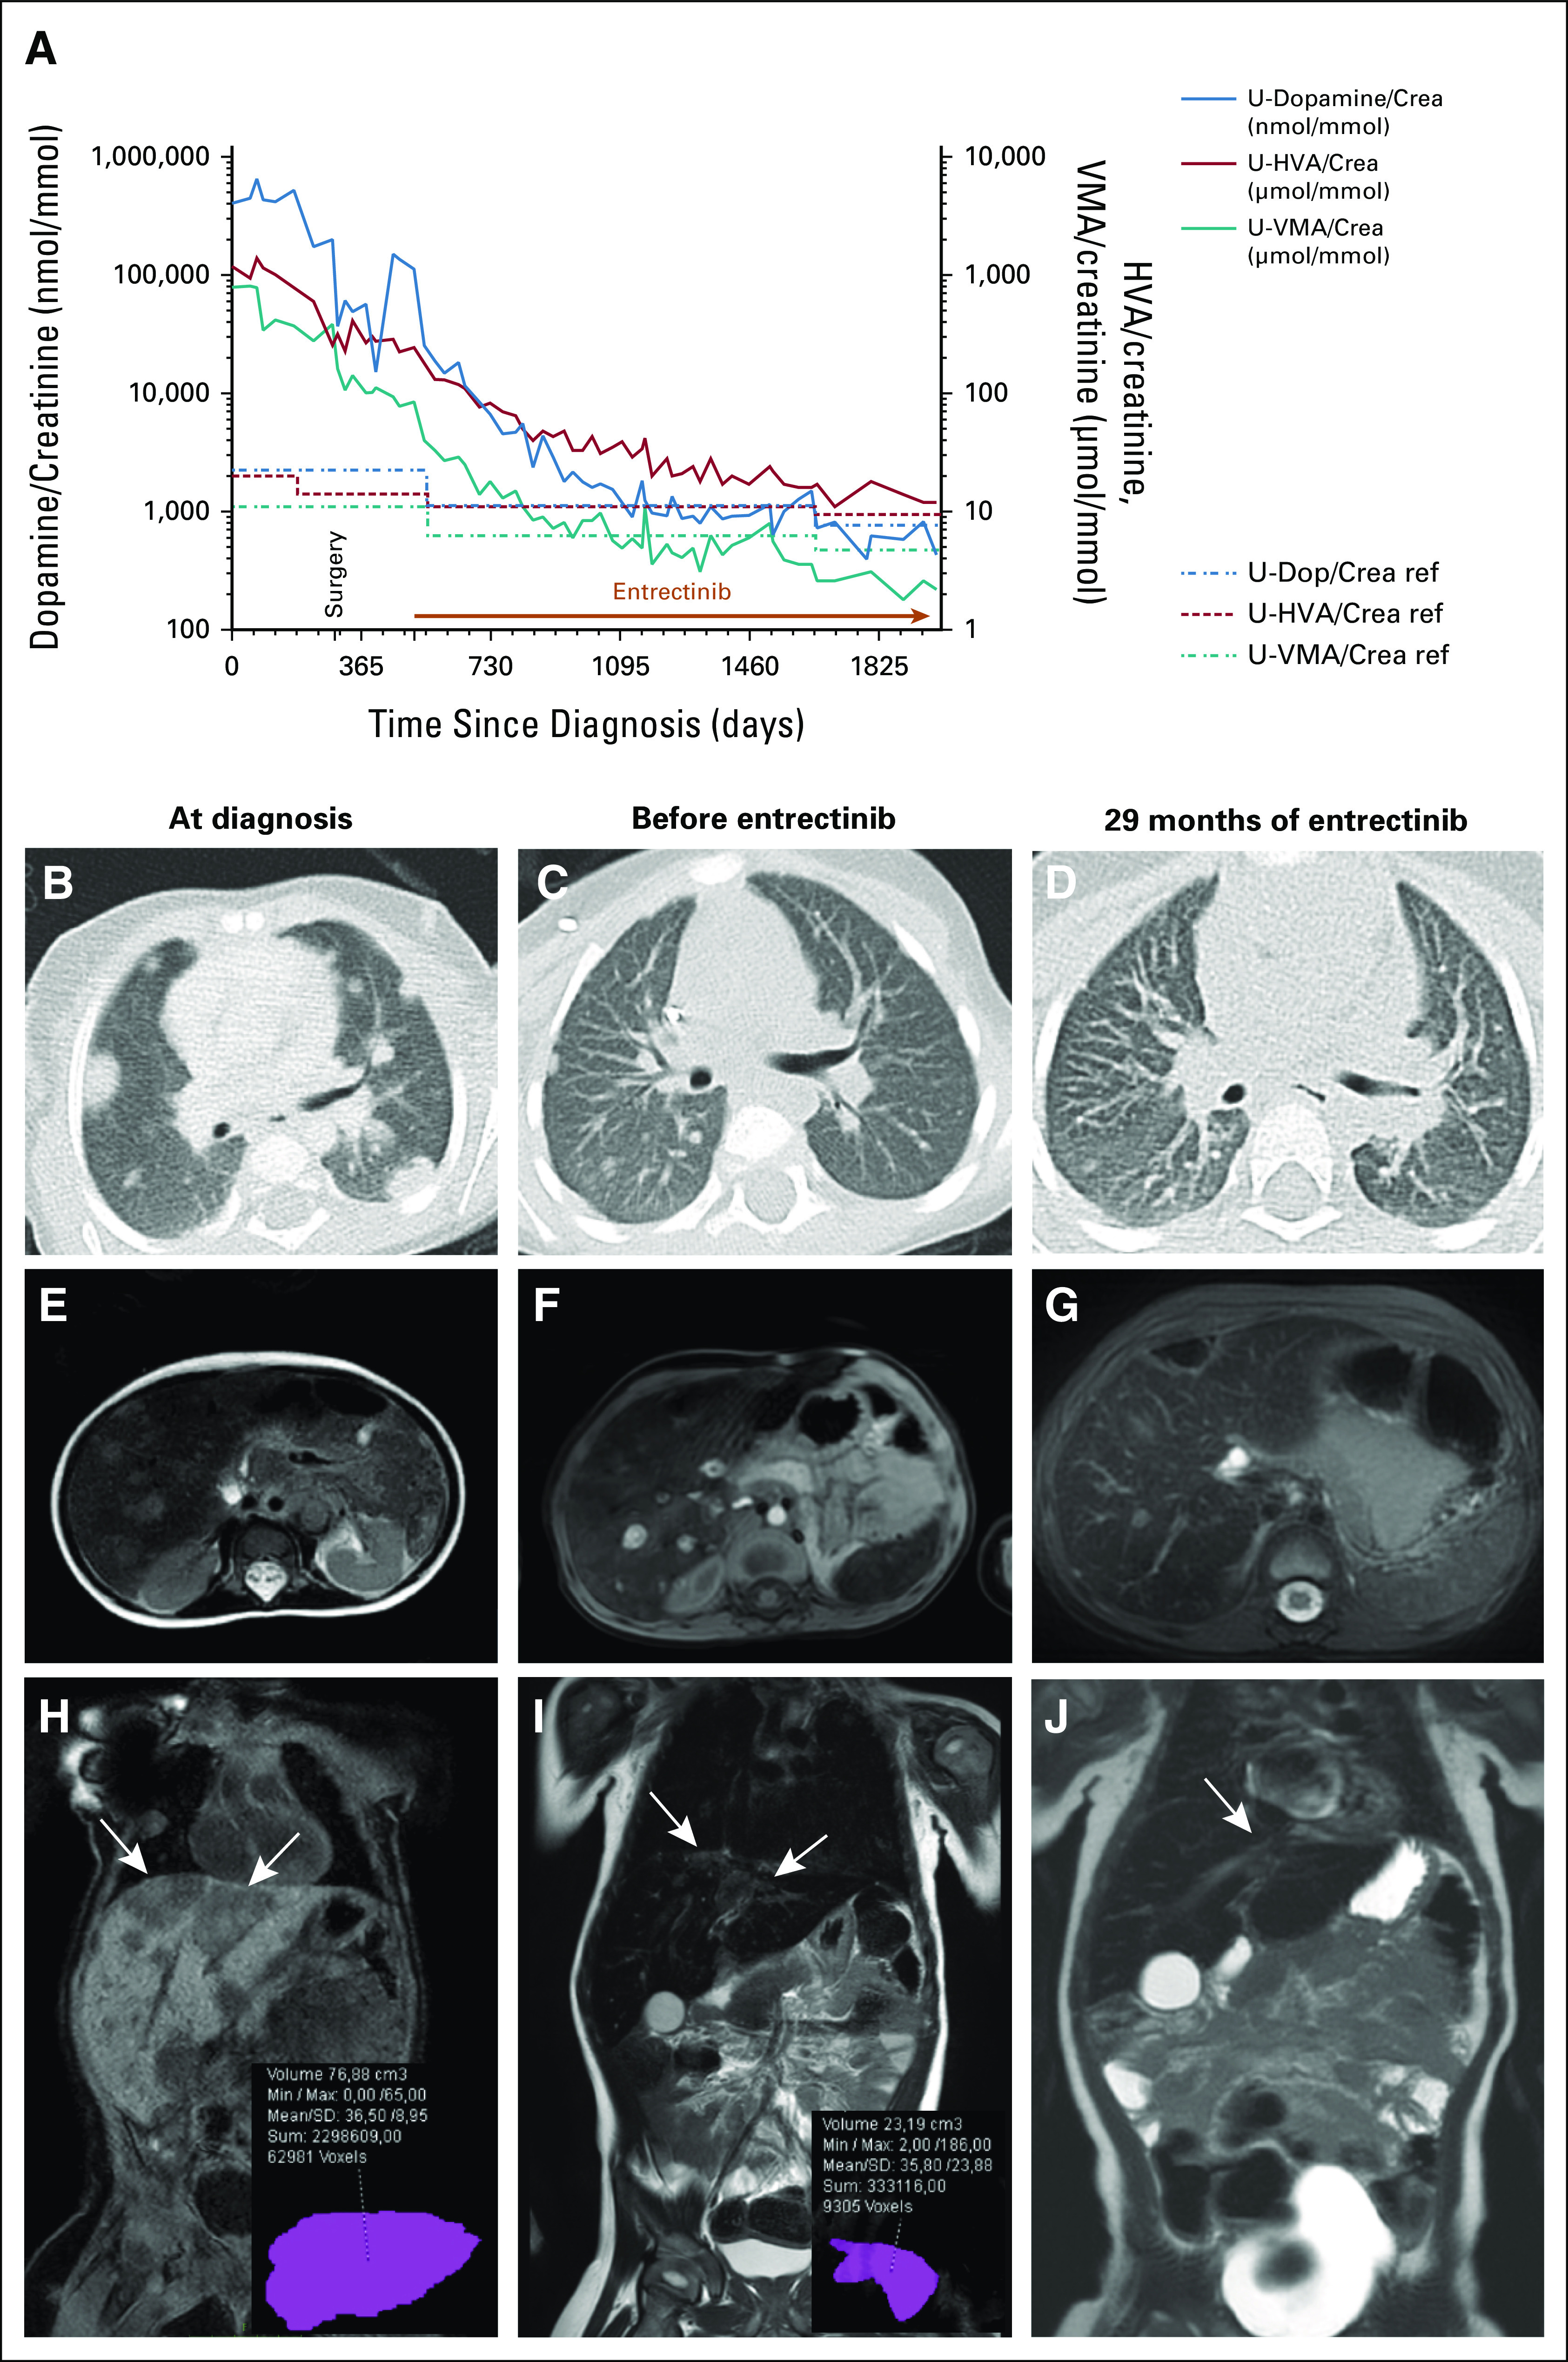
Response to treatment. (A) Urine catecholamine metabolites, logarithmic scale. Catecholamine metabolites (dopamine in blue, HVA in red, and VMA in teal) as NB markers in the patient's urine (molar concentrations/creatinine concentration). Dashed lines represent age-specific reference values. At start of entrectinib treatment, the urine dopamine level was still elevated 50-fold beyond the reference level. CT scans of the lungs showing (B) extensive metastasis at diagnosis, (C) decreasing size and number of metastases after multiple courses of chemotherapy, and (D) further involution after 29 months of entrectinib therapy. Abdominal MR images showing liver metastases (E and H) at diagnosis, (F and I) before start, and (G and J) after 29 months of entrectinib treatment. The metastasis indicated by arrows has been chosen for volumetric analysis [inserts] yielding 76 mL (H) at diagnosis, (I) 23 mL before start of entrectinib, and (J) 0 mL (unmeasurable) after 29 months of entrectinib treatment. MR image acquisition settings are as follows: (E) T2, (F) T1 + gd, (G) T1 + gd (GRE) dixon, (H) T1, (I) T2, and (J) T2. CT, computed tomography; HVA, homovanillic acid; MR, magnetic resonance; NB, neuroblastoma; VMA, vanillylmandelic acid.
